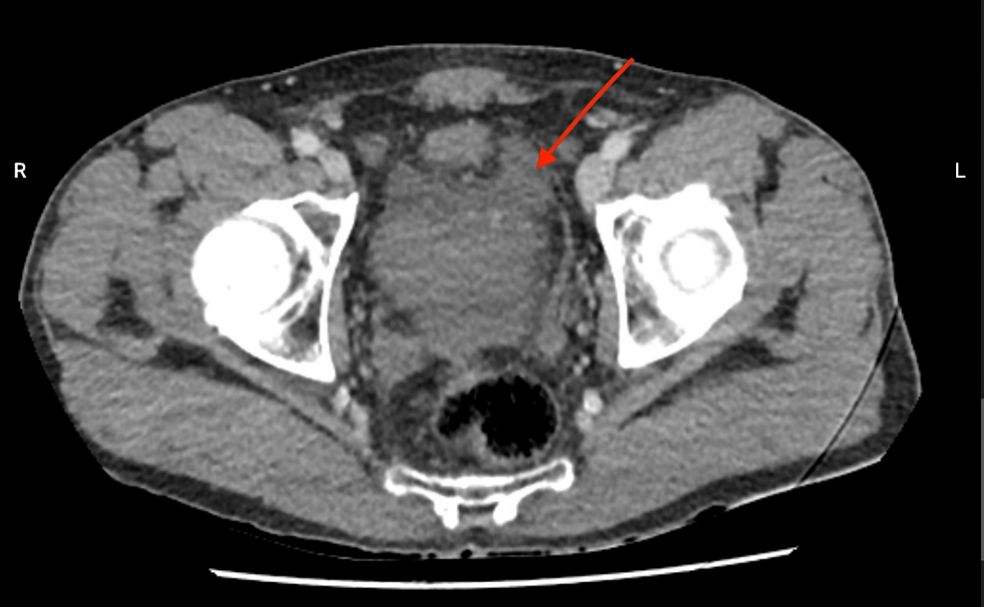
Abdominal CT scan. CT scan showing liquid in the peritoneal space in the pelvis (red arrow).
